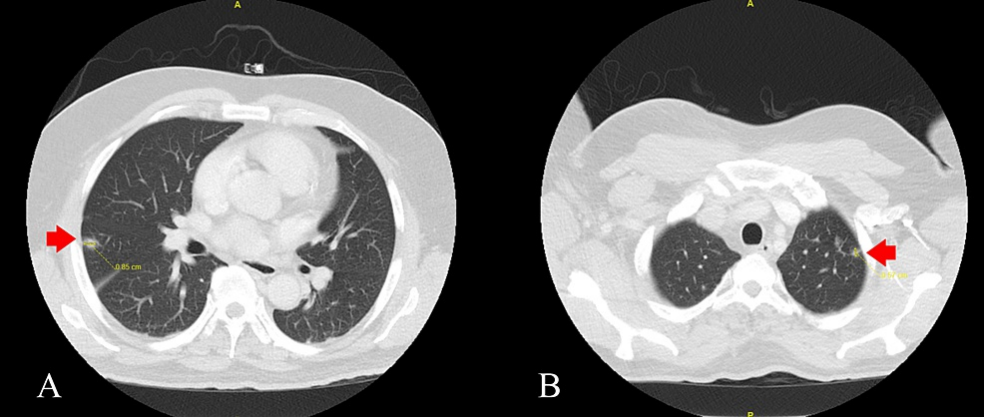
A and B: Computed tomography scan of the chest showing septic emboli in the right and left lung (red arrows)
